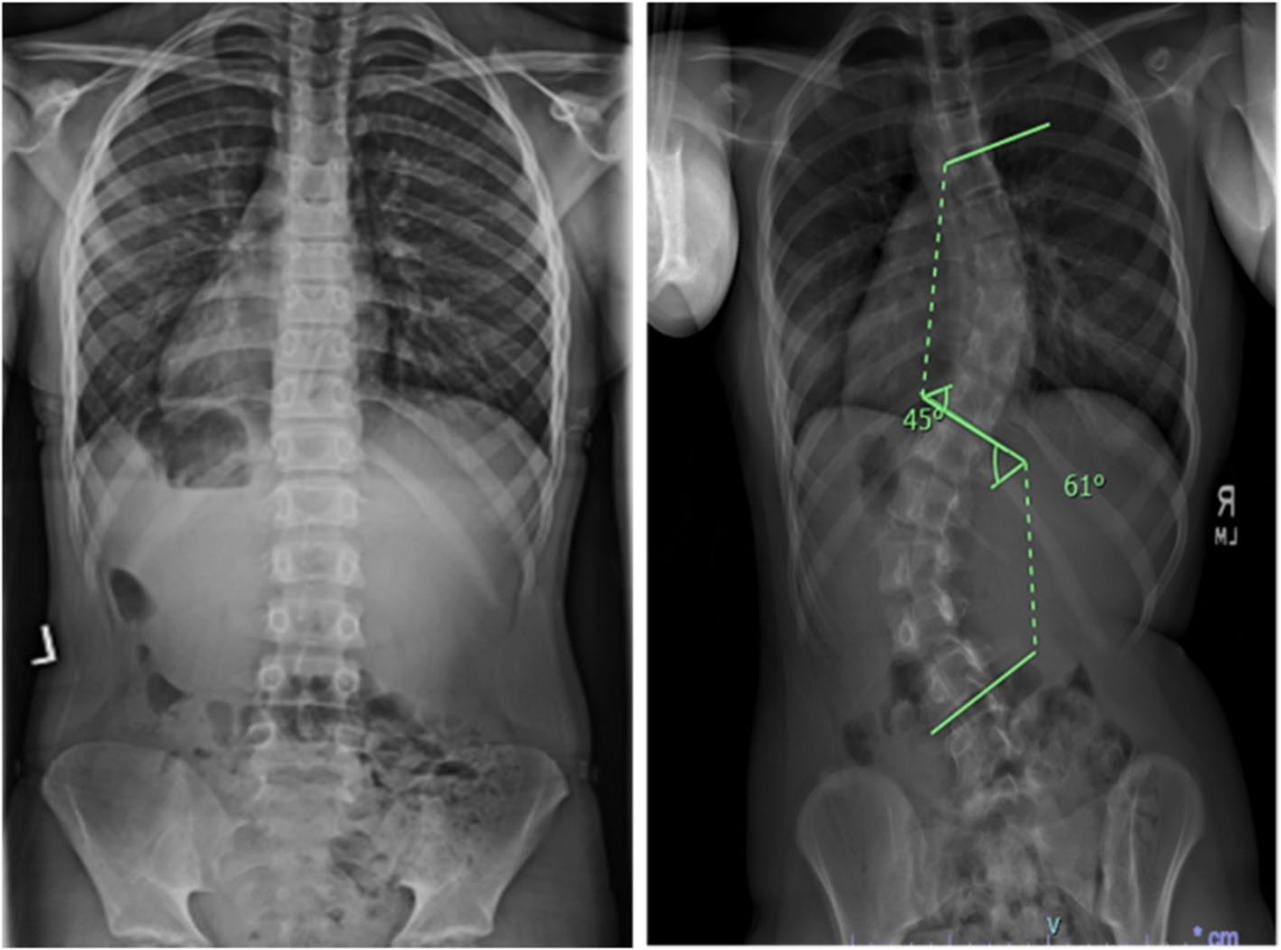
Radiographs of children with a normal spine (left) and severe adolescent idiopathic scoliosis (AIS) (right), shown with Cobb angle measurements
